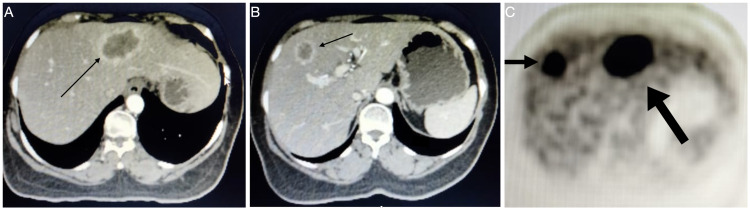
PET-CT images at initial presentation, (A) Metastatic lesion in the segment II/III of the liver (line arrow) which required a left lateral segmentectomy, (B) Metastatic lesion in the segment IVa/VIII of the liver (line arrow), which was ablated, and (C) PET image demonstrating FDG uptake in both the lesions in segment II/III (long block arrow) and segment IVa/VIII (short block arrow). PET: Positron emission tomography; FDG: Fluorodeoxyglucose.
